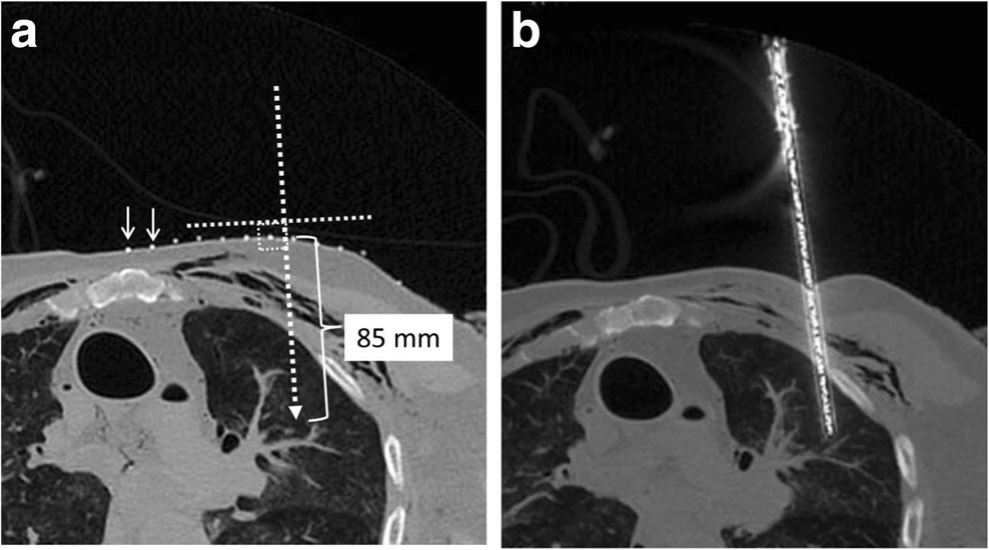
a The radio-opaque skin marker strip is placed on the left chest wall (*small arrows*), and the angle of approach and depth of insertion of the needle can be planned using the geometry tools on the CT workstation. The needle is then be inserted at the planned angle and distance and a further set of CT images (b) are acquired to confirm the location of the needle prior to biopsy
